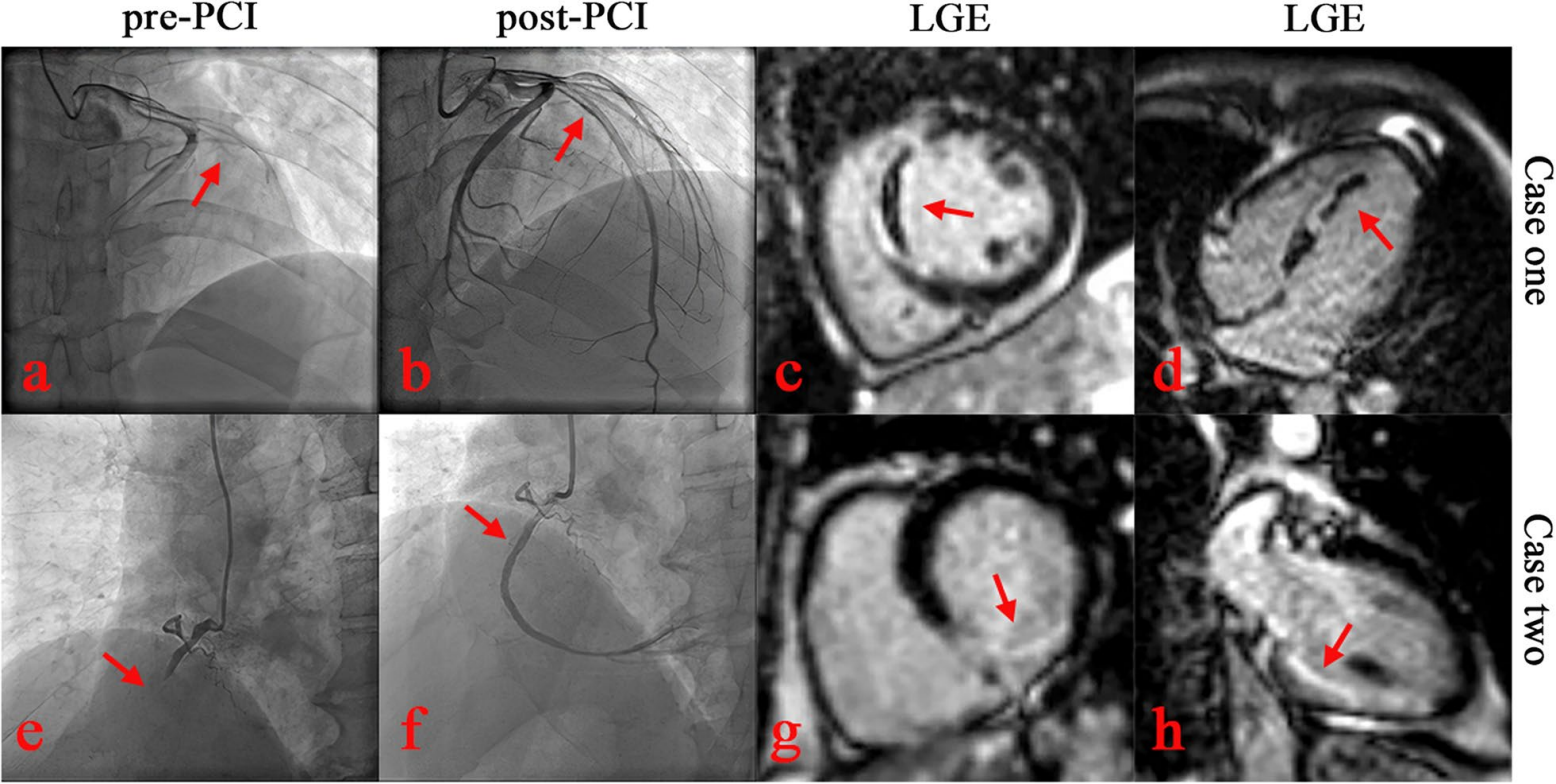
Two typical examples (with and without MVO) of angiography and cardiac magnetic resonance image. Case one (a–d): A 49-year-old male STEMI patient with MVO was diagnosed to have a total occluded proximal segment of the LAD (SYNTAX score 20.5, SYNTAX II score 39, and Gensini score 80); Case two (e–h): A 68-year-old male STEMI patient without MVO was diagnosed to have a total occluded proximal segment of the RCA (SYNTAX score 10, SYNTAX II score 30.6, and Gensini score 32). *STEMI* ST-segment elevation myocardial infarction; *MVO* microvascular obstruction; *LAD* left anterior descending artery; *RCA* right coronary artery
